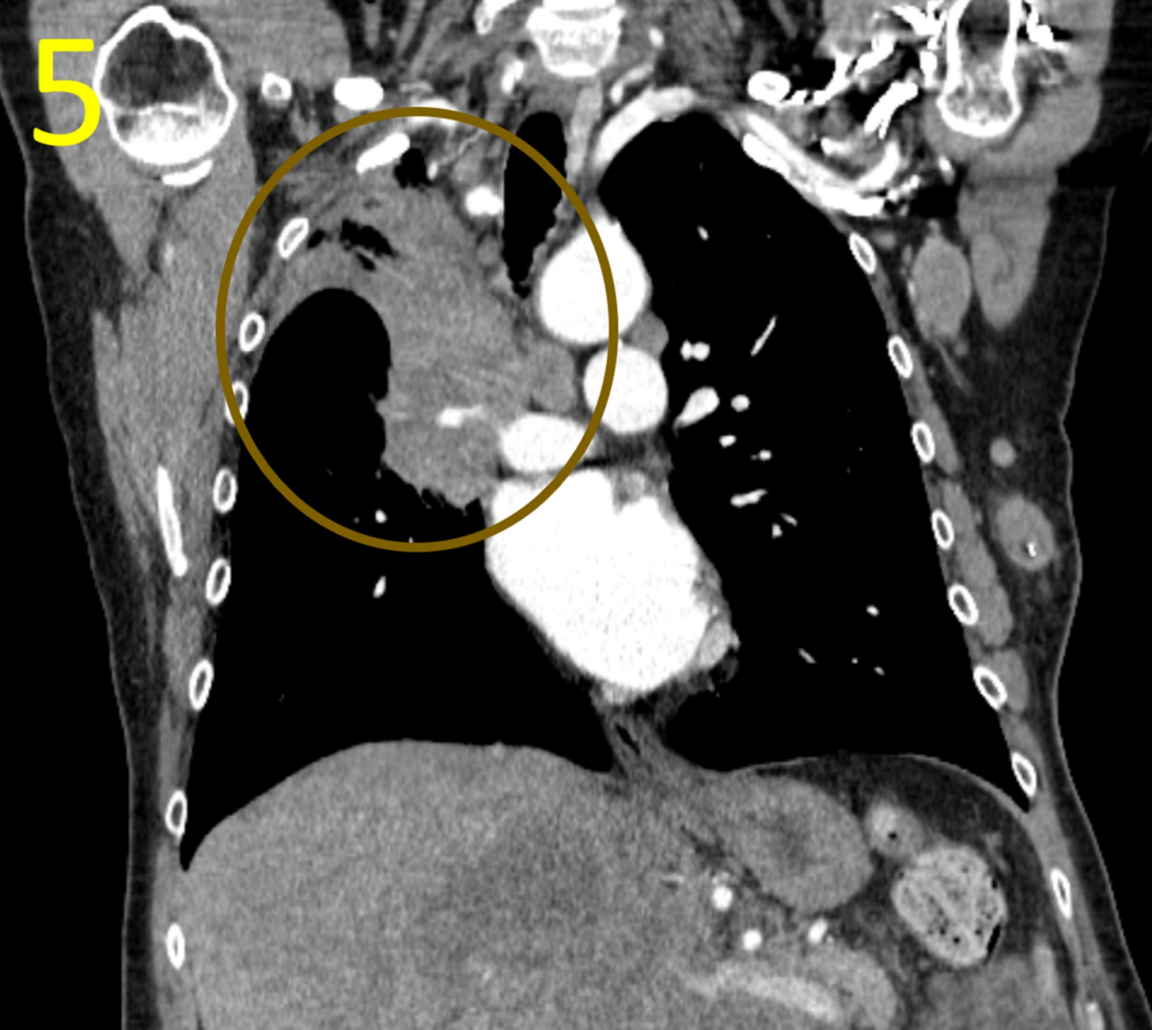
Coronal Reconstruction of Chest CT Scan This coronal reconstruction CT scan demonstrates a large right hilar mass (brown circle) causing complete collapse of right upper lobe. This mass is the likely primary site of this patient's neuroendocrine small cell lung cancer.
